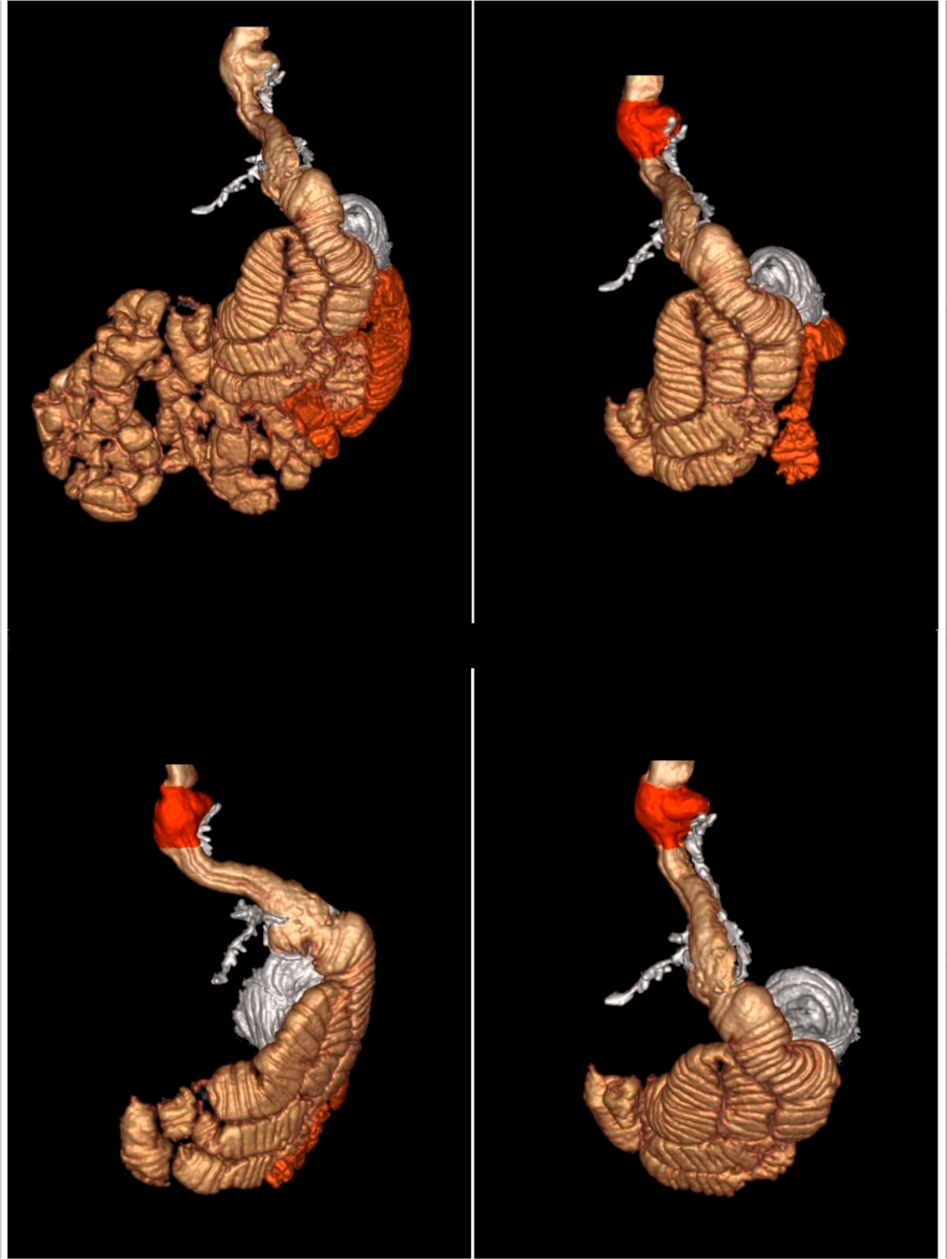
CT 3D volumetry showing a patient post-laparoscopic sleeve gastrectomy (LSG) and Roux-en-Y gastric bypass (RYGB) as primary surgeries, with a sliding hiatus hernia
